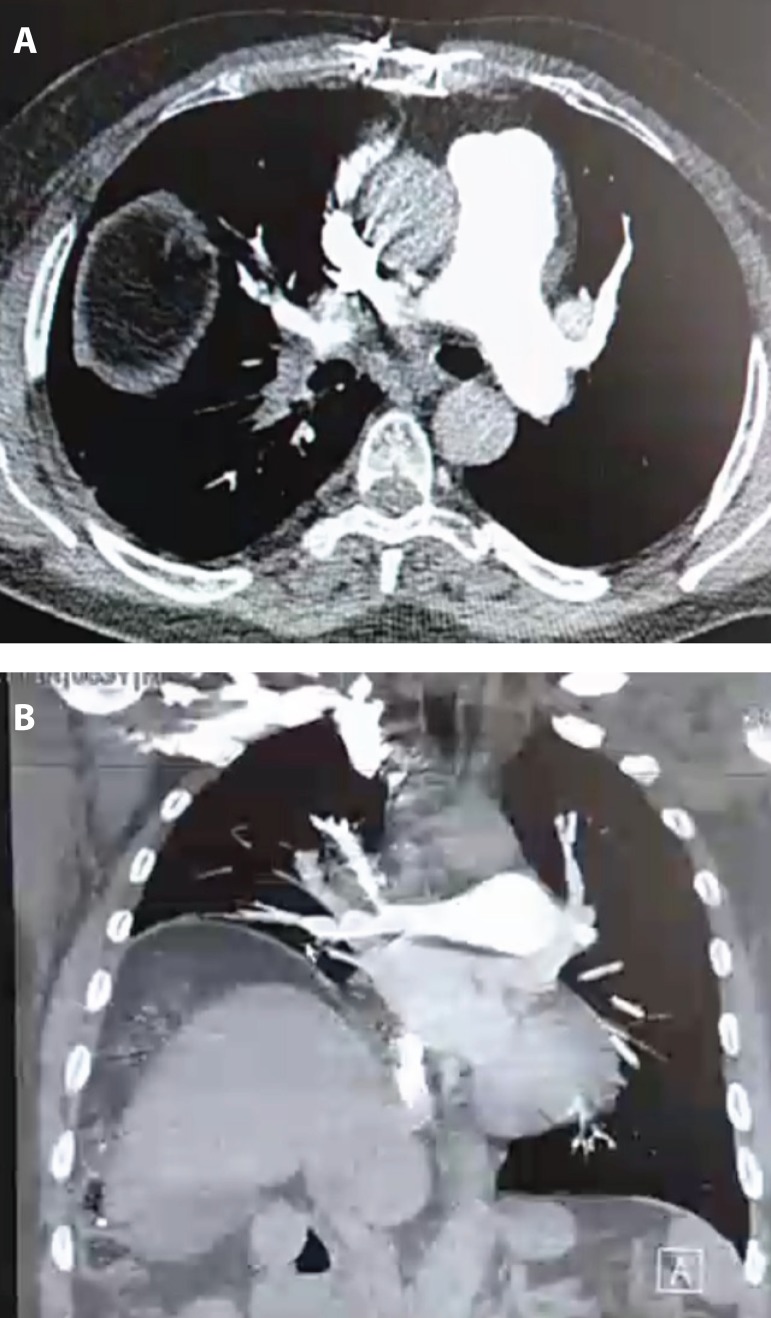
(A). CT scan showing complete thromboembolic obstruction of the right lower lobe and (B). Elevation of the right diaphragm.
